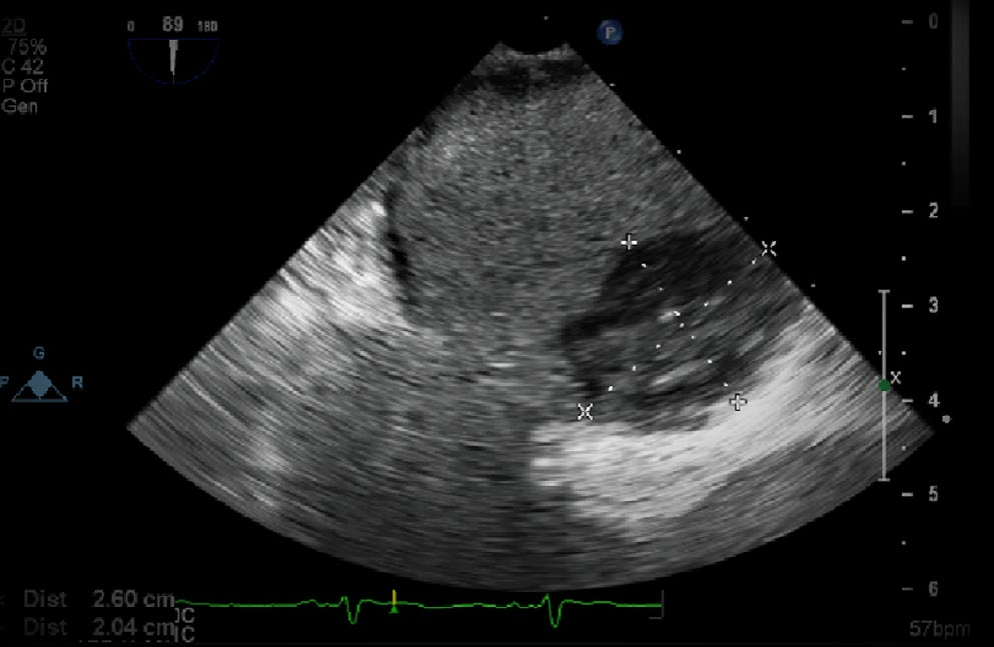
is showing a transoesophageal echocardiogram with evidence of huge left atrial thrombus
